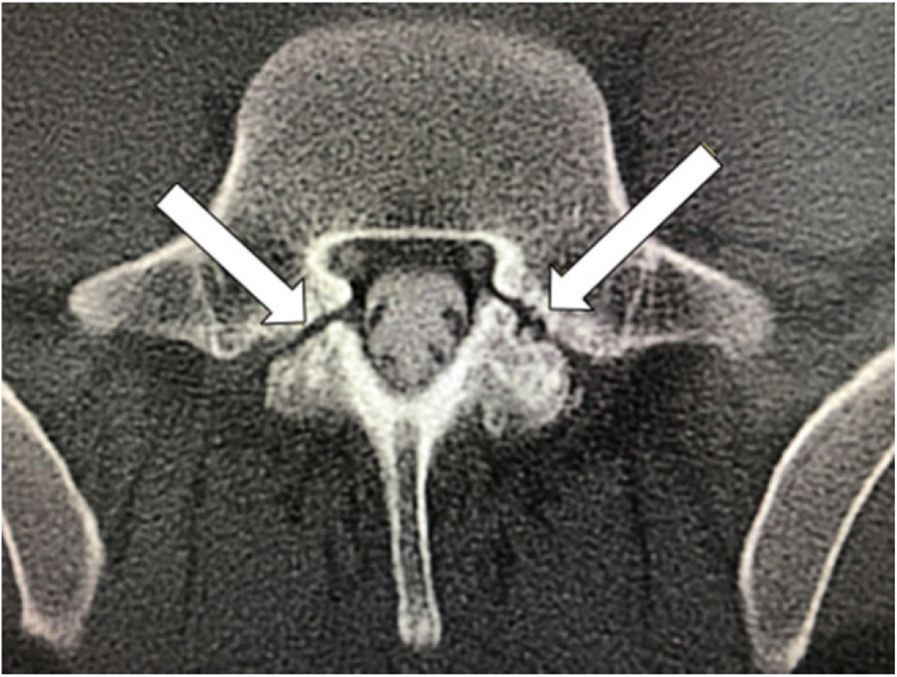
Computed Tomography Myelogram (axial view) demonstrated bilateral L5 pars interarticularis fractures (arrows) (Patient 1)
